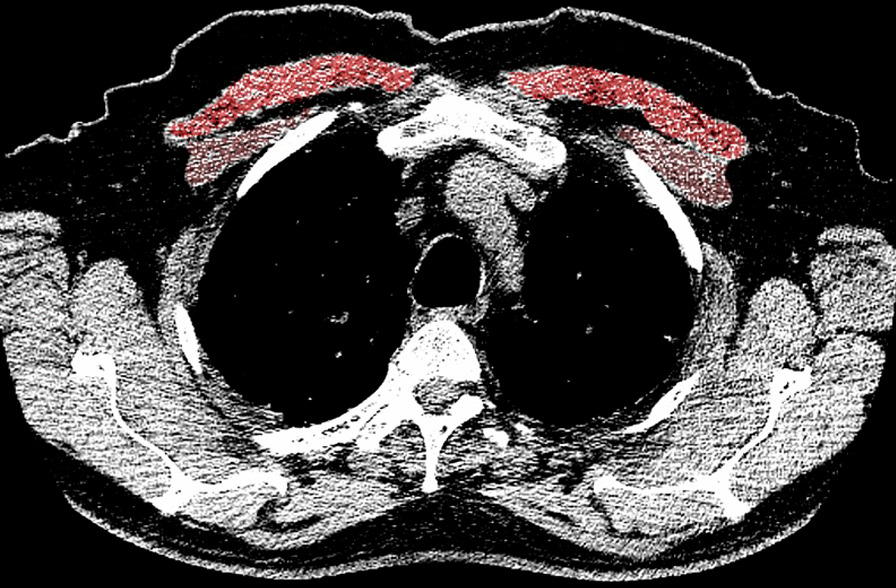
Measurement of pectoralis major and minor cross-section areas at T4 vertebra, using CT slicer
